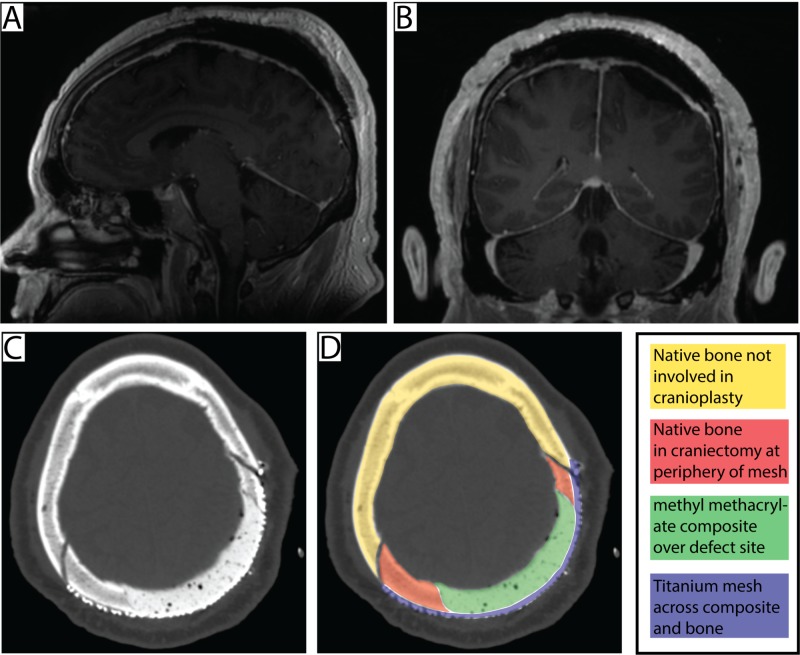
Postoperative imaging. A: Sagittal and B: coronal postcontrast T1 weighted postoperative MR image showing gross total resection of the metastatic lesion. C: bone weighted postoperative CT scan showing the cranioplasty site. D: The different areas of bone involved in the cranioplasty are shown color coded based on the image in C.
